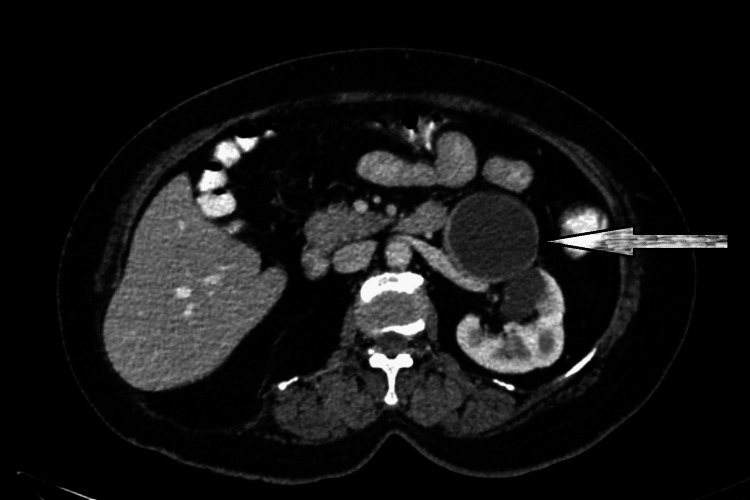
CT with contrast, axial section abdomen showing left side pararenal and renal space occupying lesion (SOL) A CT scan showed a peripherally enhanced hypoattenuating large cystic left adrenal mass in the retroperitoneum and along the inferolateral aspect of the left adrenal gland, seen abutting the pancreatic tail (arrow). Metastatic deposits or masses were not found.
